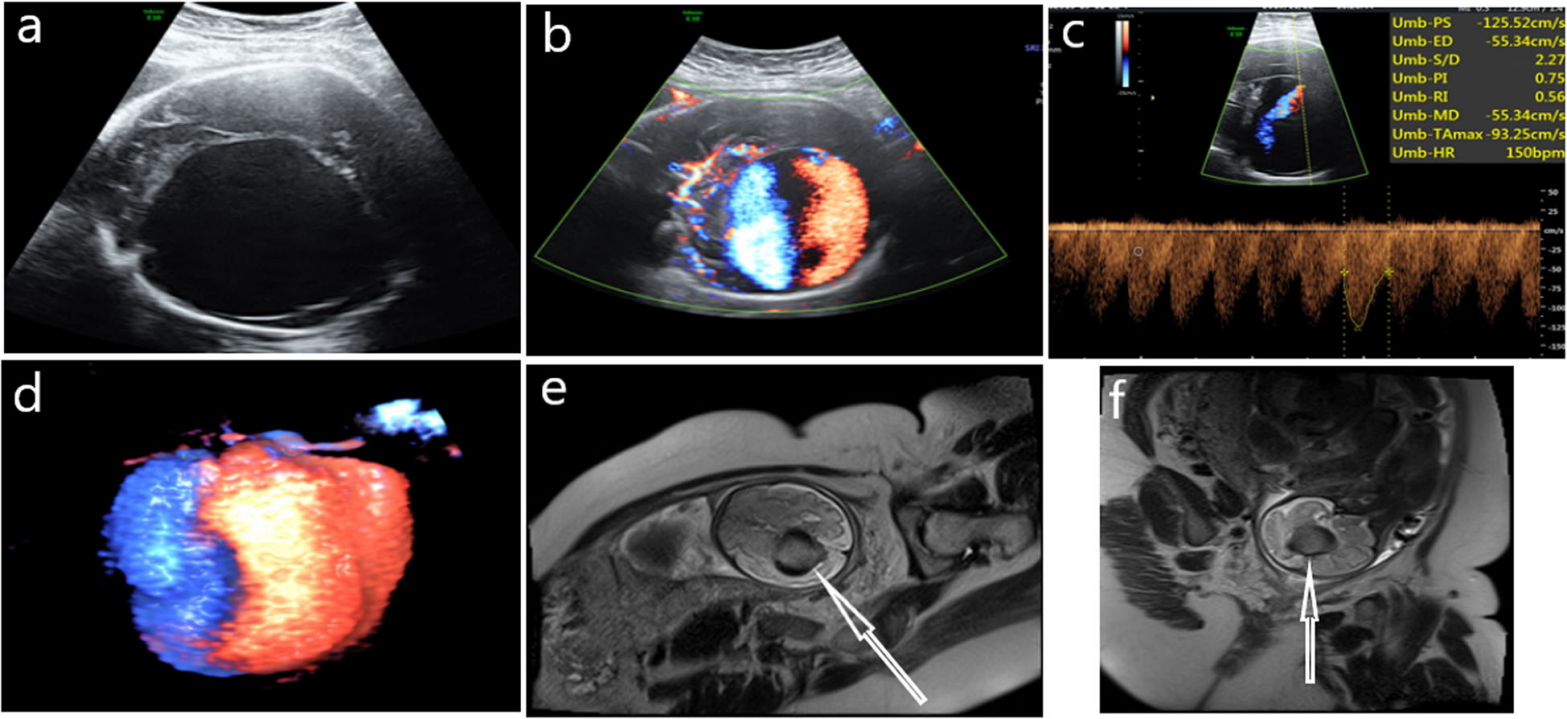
Case 1, 35-week prenatal ultrasound and MRI images of a VGAM foetus. a: Two-dimensional ultrasound shows a huge thin-walled echoless area next to the midline of the brain. b: Colour Doppler shows a full blood-flow signal in the brain’s echoless area. c: The skull’s echoless area shows a high-speed low-impedance blood-flow spectrum. d: The reconstructed three-dimensional energy Doppler image clearly shows the dilated Galen vein, but no obvious straight sinus is displayed. e: MRI cross-section of the foetal skull showing the dilated Galen vein through flow phenomenon (white arrow). f: Sagittal section of the foetal brain showing dilated Galen’s venous aneurysm by flow phenomenon (white arrow)
